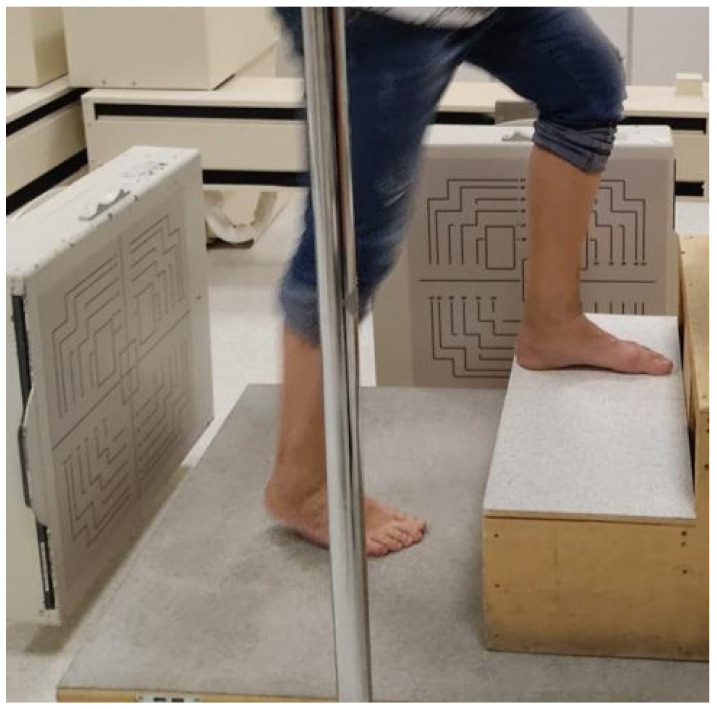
Radio-stereometric fluoroscopy, showing orthogonally positioned X-ray detectors while climbing a step.
